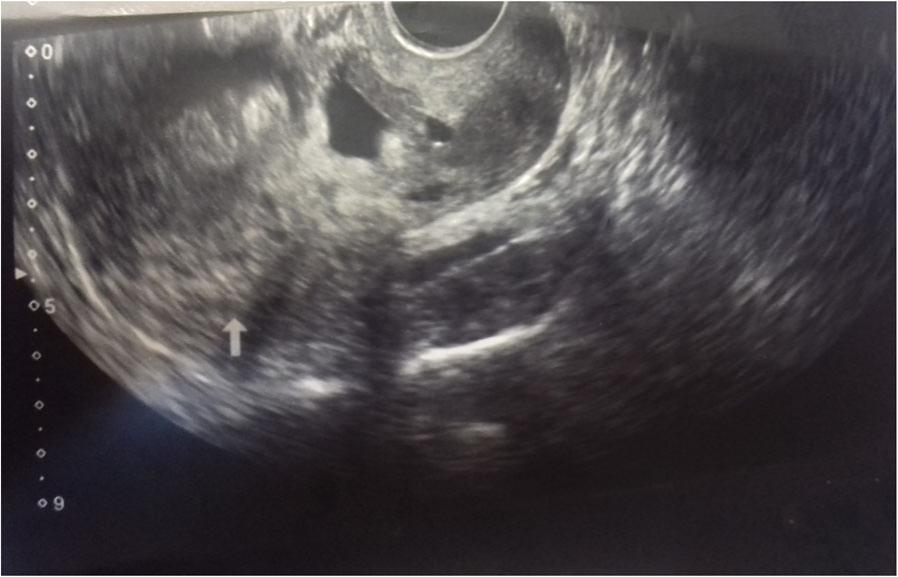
Transvaginal ultrasound scan longitudinal view
